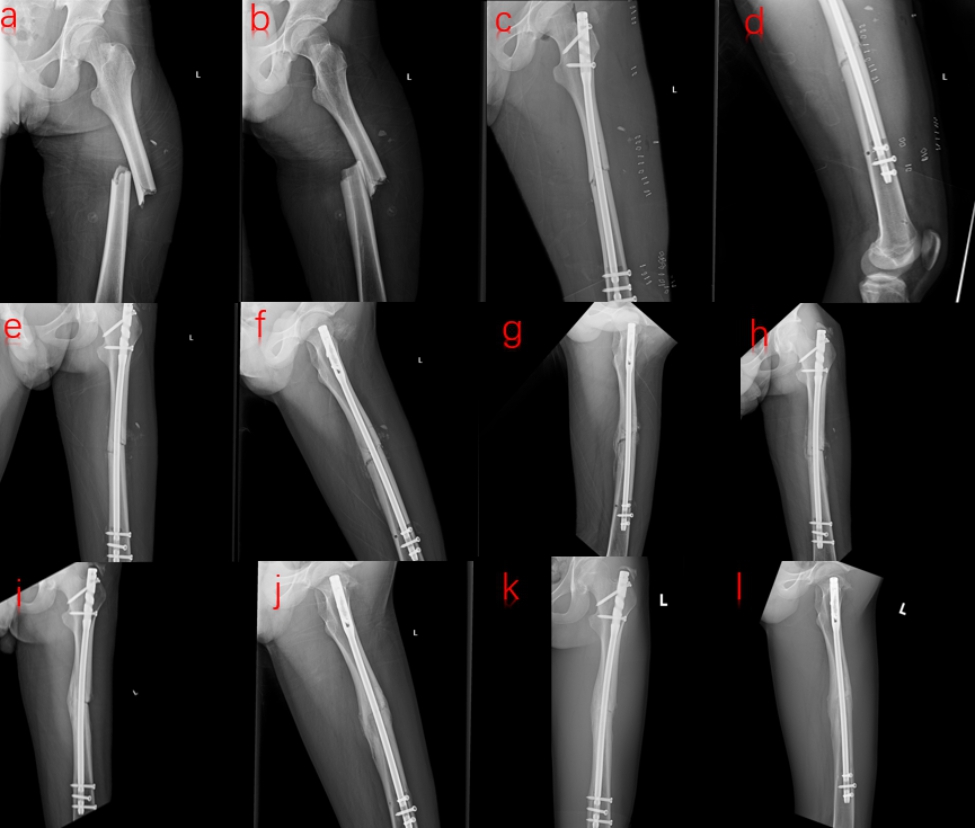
Radiographs of a case after DRTR: (a, b) Preoperative anterior-posterior (AP) and lateral views; (c, d) AP and lateral views on the second postoperative day; (e, f) AP and lateral views one month postoperatively; (g, h) AP and lateral views three months postoperatively; (i, j) AP and lateral views 6 months postoperatively; (k, l) AP and lateral views 12 months postoperatively
